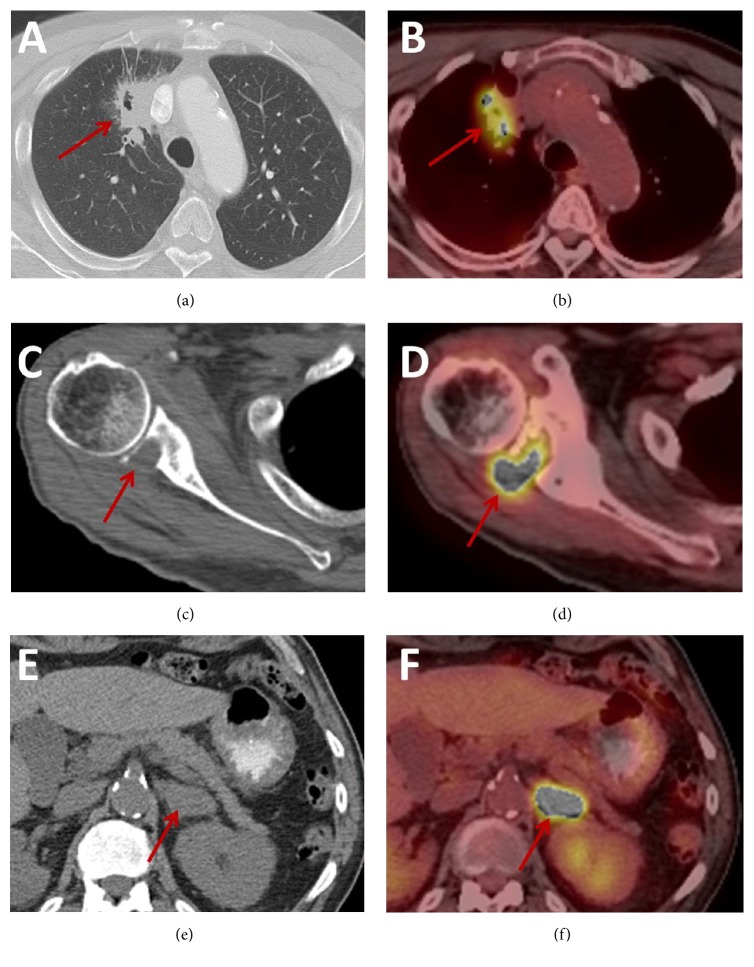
Axial noncontrast CT image in the lung window (a) demonstrates a spiculated mass with an area of central cavitation in the right upper lobe abutting the mediastinum (solid arrow) with imaging features suggesting a primary pulmonary squamous cell carcinoma. The corresponding PET/CT image (b) demonstrates hypermetabolic activity (solid arrow) within this lesion. Axial noncontrast CT image in the bone window (c) demonstrates a soft tissue lesion centered in the right subscapularis muscle with destruction of the adjacent bony glenoid (arrow). Corresponding PET/CT imaging (d) demonstrates hypermetabolic activity (solid arrow) within this lesion. Axial noncontrast CT image in the soft tissue window (e) demonstrates a solid lesion in the left adrenal gland (solid arrow) with the corresponding PET/CT image (f) demonstrating hypermetabolic activity (solid arrow) within this lesion.
